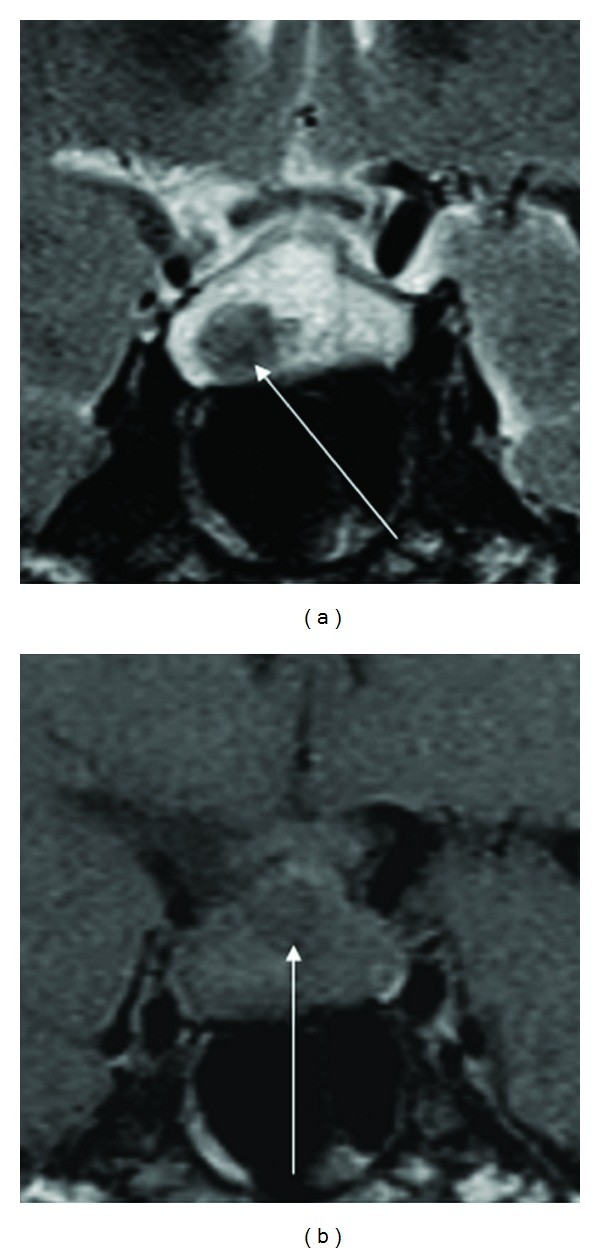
Case 17: nonenhanced coronal MRI images of RCC. (a) T2-weighted image: nodule with hypointense signal at right lower part (arrow). (b) T1-weighted image: nodule with hypointense signal at the upper part (arrow).
